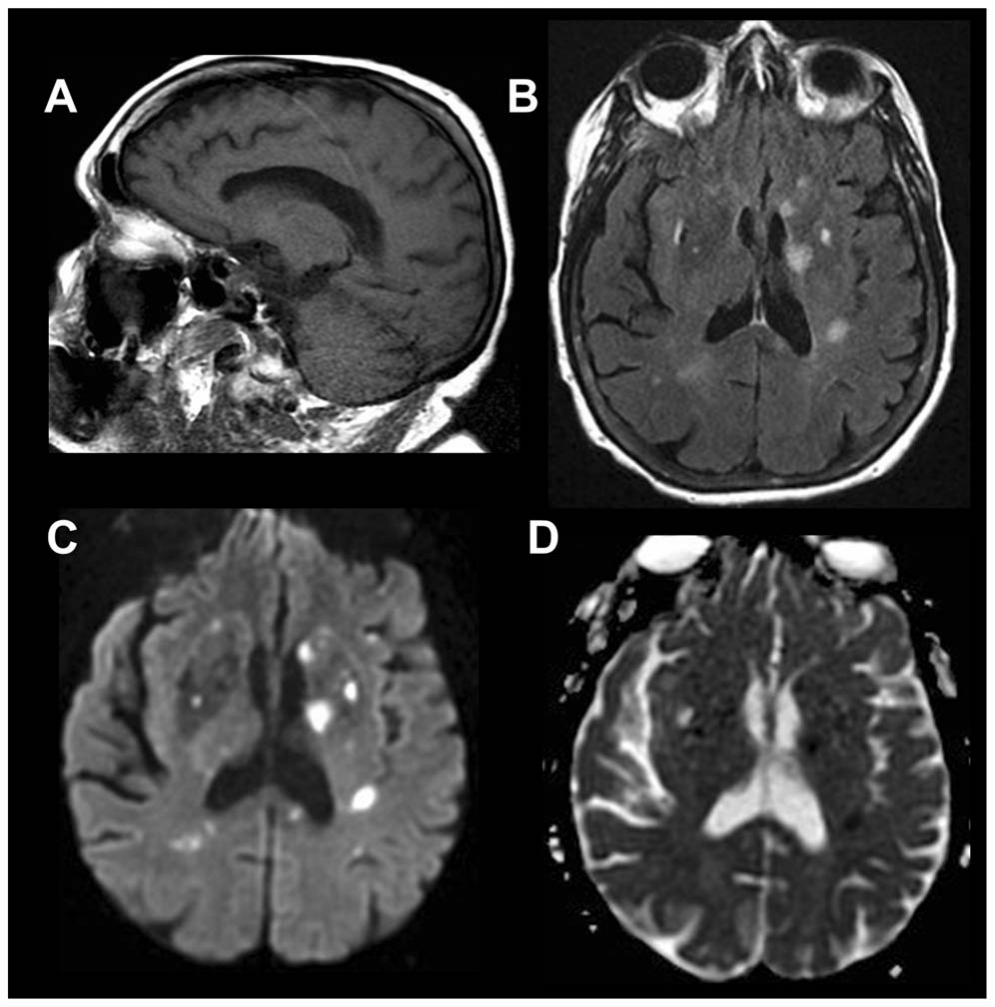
Acute infarctions in a 77 year old female with altered mental status. A 77-year-old woman underwent MRI examination because of altered mental status. Her complete examination included 12 pulse sequences, but all significant abnormalities are apparent in images derived from the three pulse sequences that comprised the abbreviated examination described in phase 1 of this study. A sagittal T1-weighted image (A) reveals no apparent abnormality. Axial T2-weighted FLAIR images (B) demonstrate multiple small hyperintense lesions in deep gray matter and white matter structures. The lesions are markedly hyperintense in diffusion-weighted images (C) and apparent diffusion coefficient maps derived from the DWI sequence (D) show restricted diffusion within the lesions, confirming that they are ischemic infarcts.
